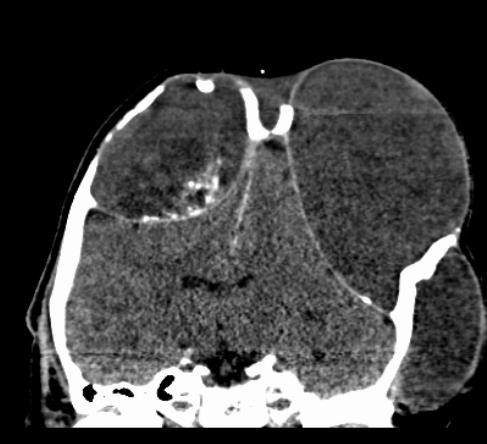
Coronal reconstruction of computed tomography (CT) scan reveals the lack of the outer table and the thinning of the inner table due to the expansive intradiploic cystic mass. Intratumoral calcification is shown on the right side.
